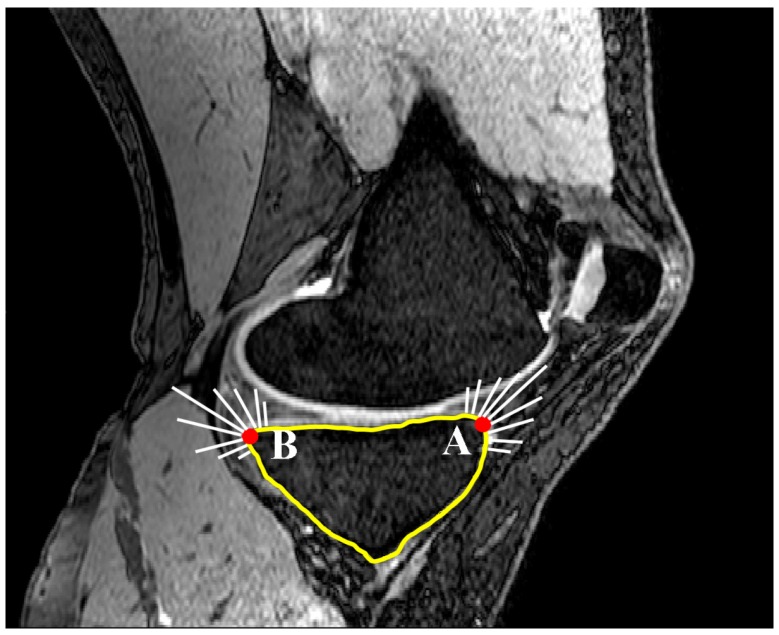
Locating nodes A and B based on Gaussian curvature analysis for each MRI slice (sagittal view).
